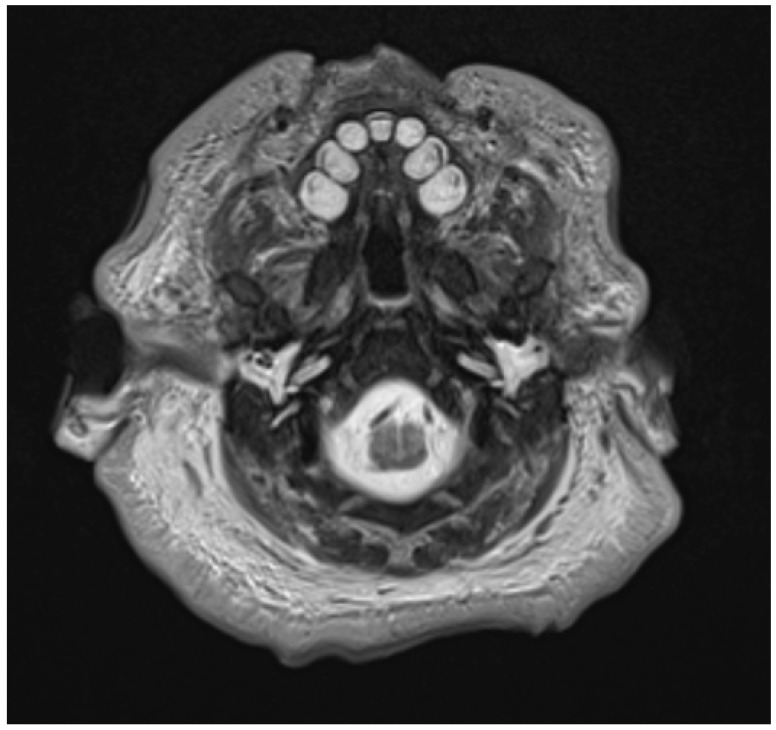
Eight-day old male with holoprosencephaly. Axial T2-weighted MRI demonstrates a single central incisor tooth germ. This appearance is associated with holoprosencephaly the diagnosis and should direct attention to potentially associated abnormalities in the brain.
